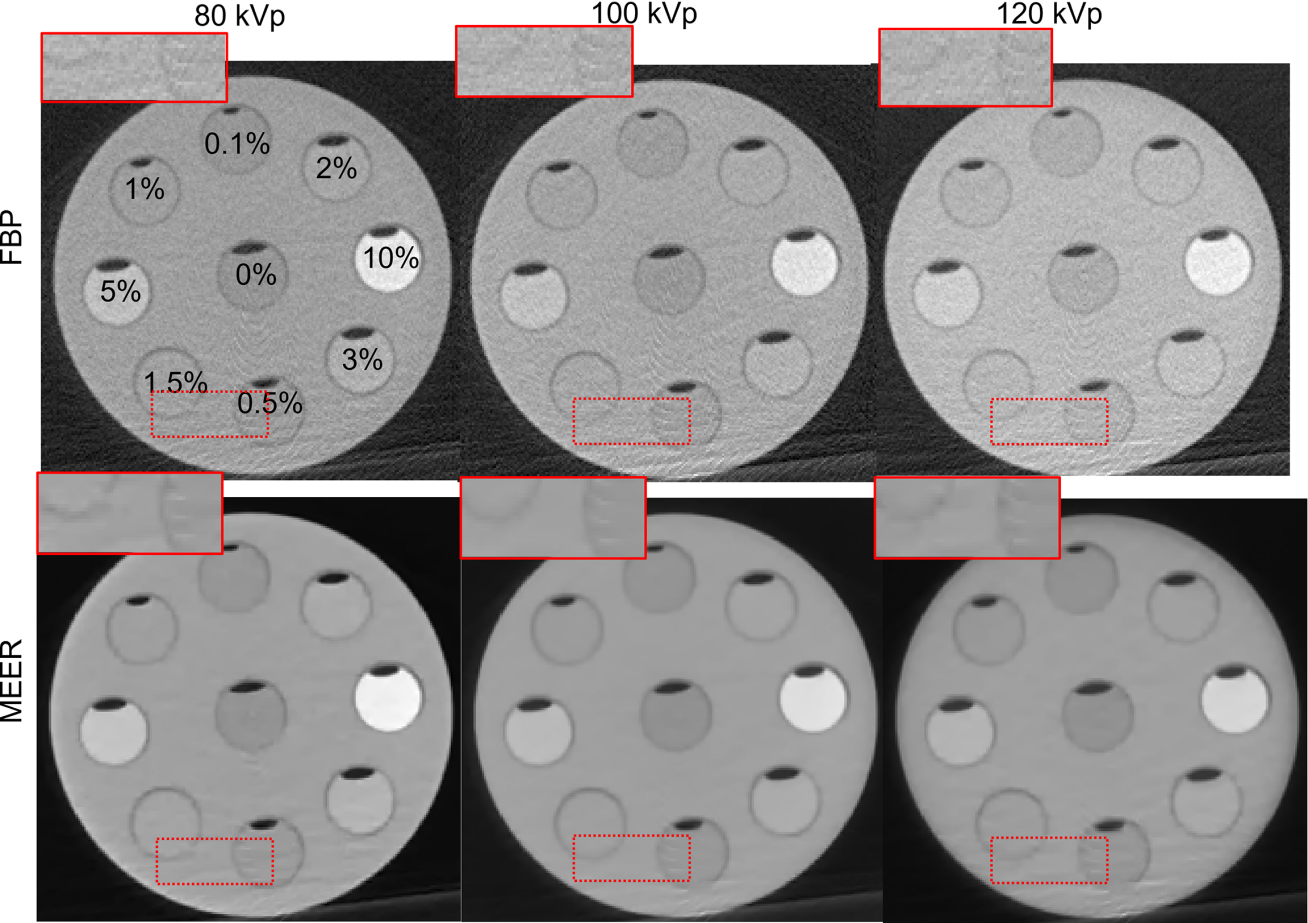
Reconstructed CT images in experimental study. Dashed rectangles indicate zoomed in view regions. The CT images are displayed at a window of [-500, 500] HU.
